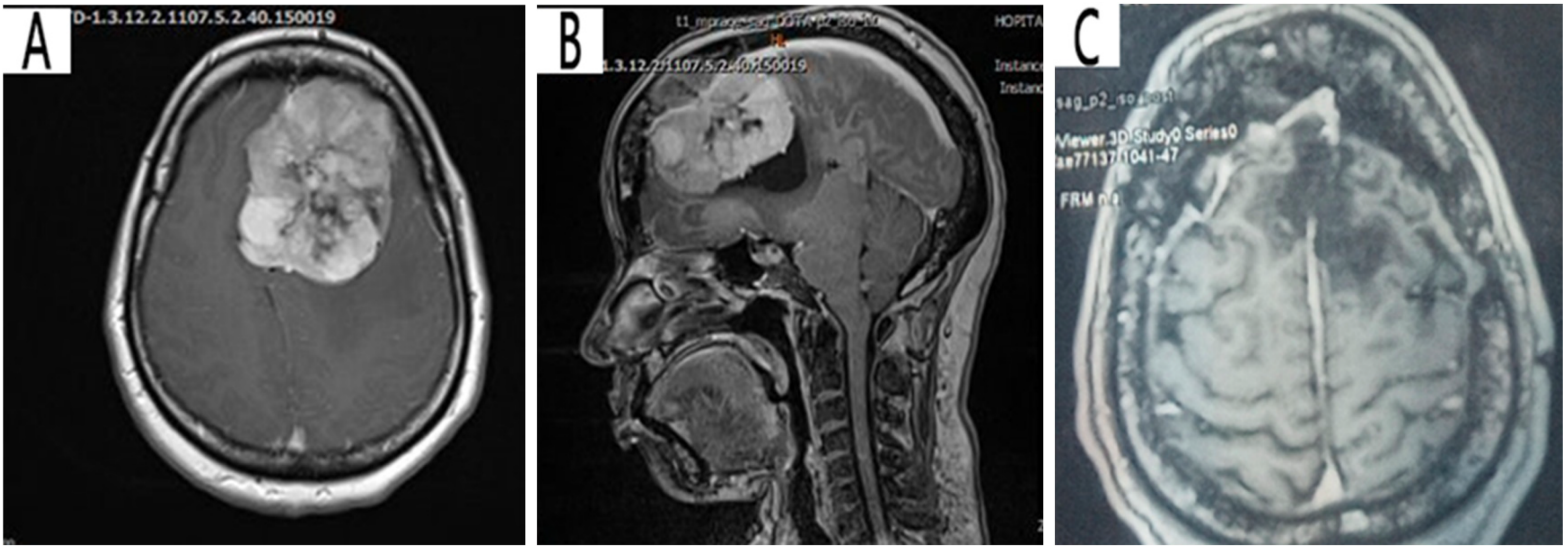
Pre-operative T1 cerebral MRI with contrast showing a left hyperintense frontal parasagittal mass with dural insertion: (A) axial view, (B) sagittal view (C) Postoperative T1 cerebral MRI showing the site of the tumor resection (porencephalic cavity).
